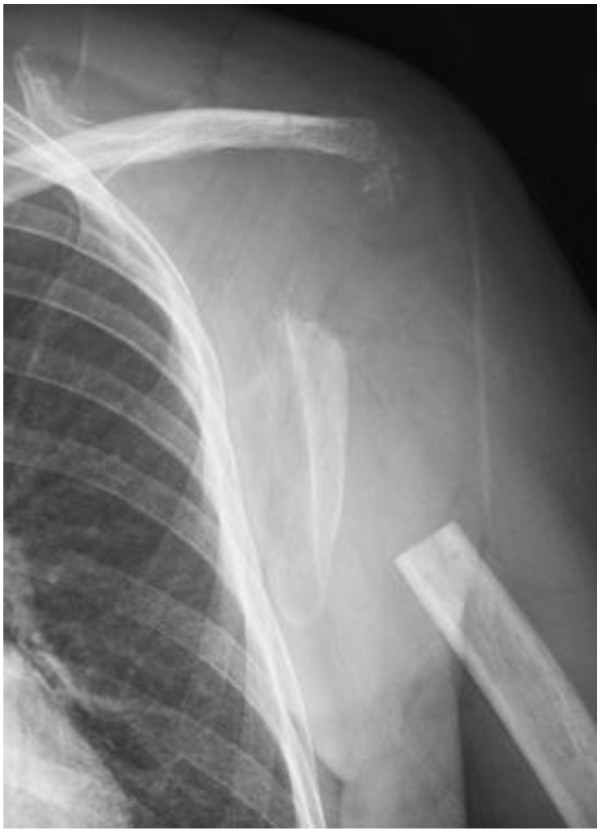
Immediate post-operative radiograph of the left shoulder after wide resection.
